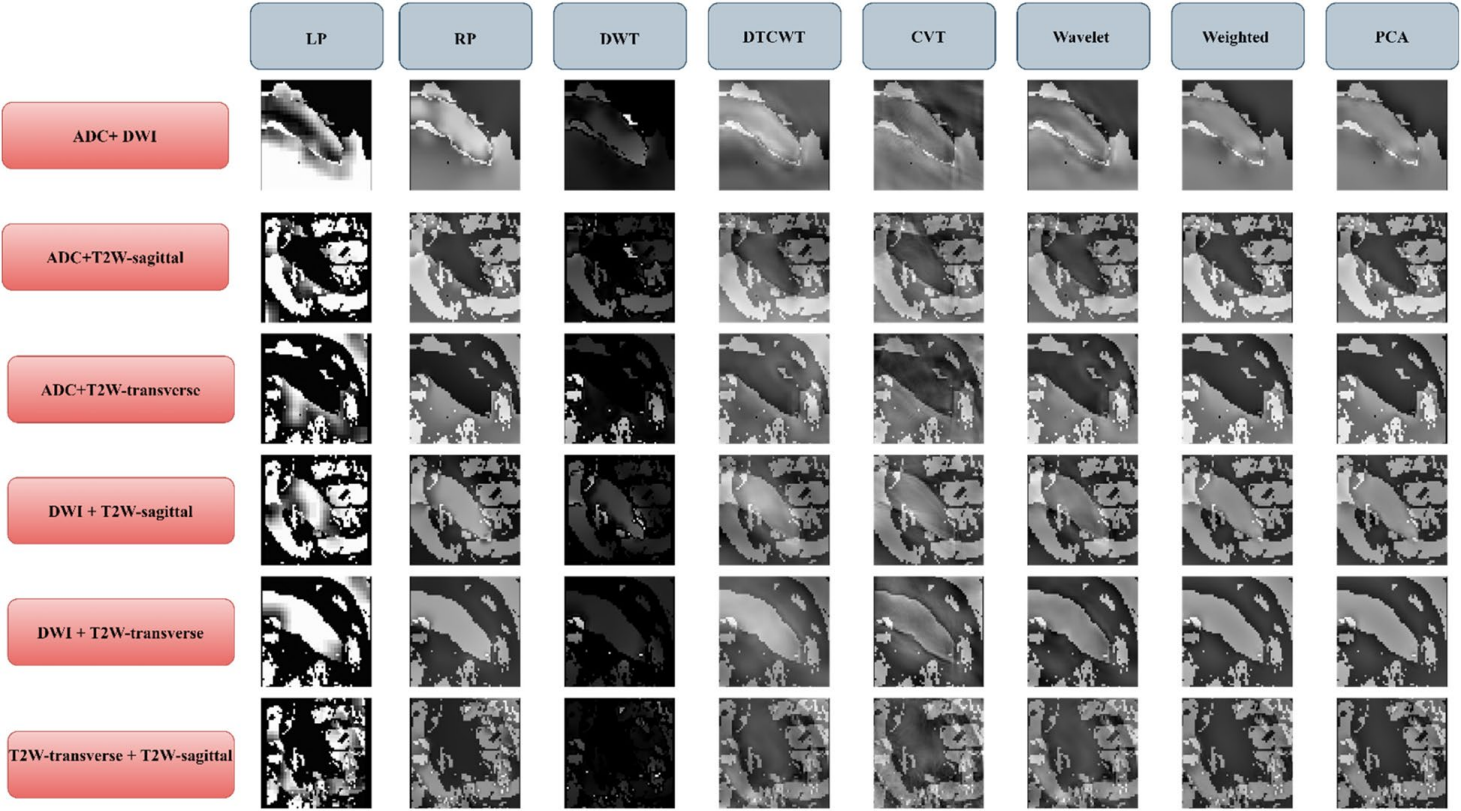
The fusion of T2W-transverse, T2W-sagittal, DWI, and ADC using 8 fusion techniques
